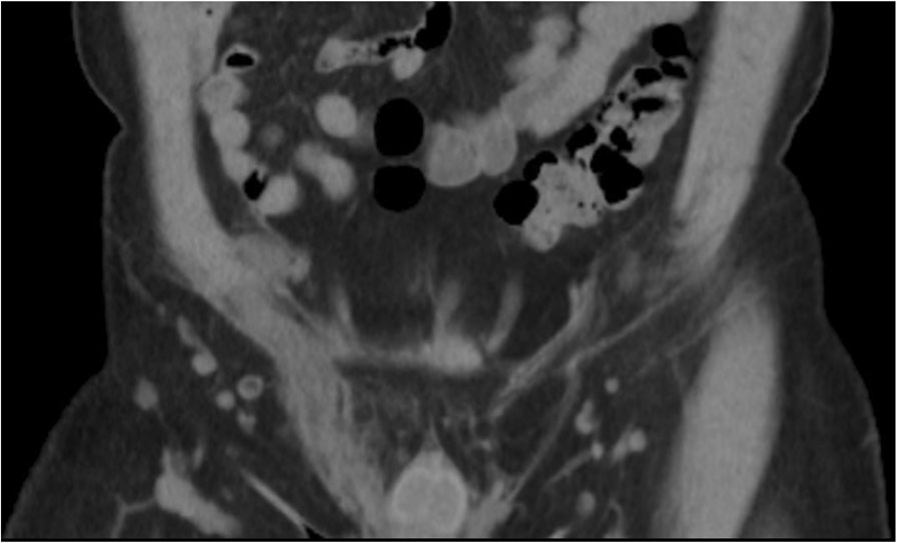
Non-enhanced coronal CT image revealed diffuse edematous change of right spermatic cord (white arrow)
